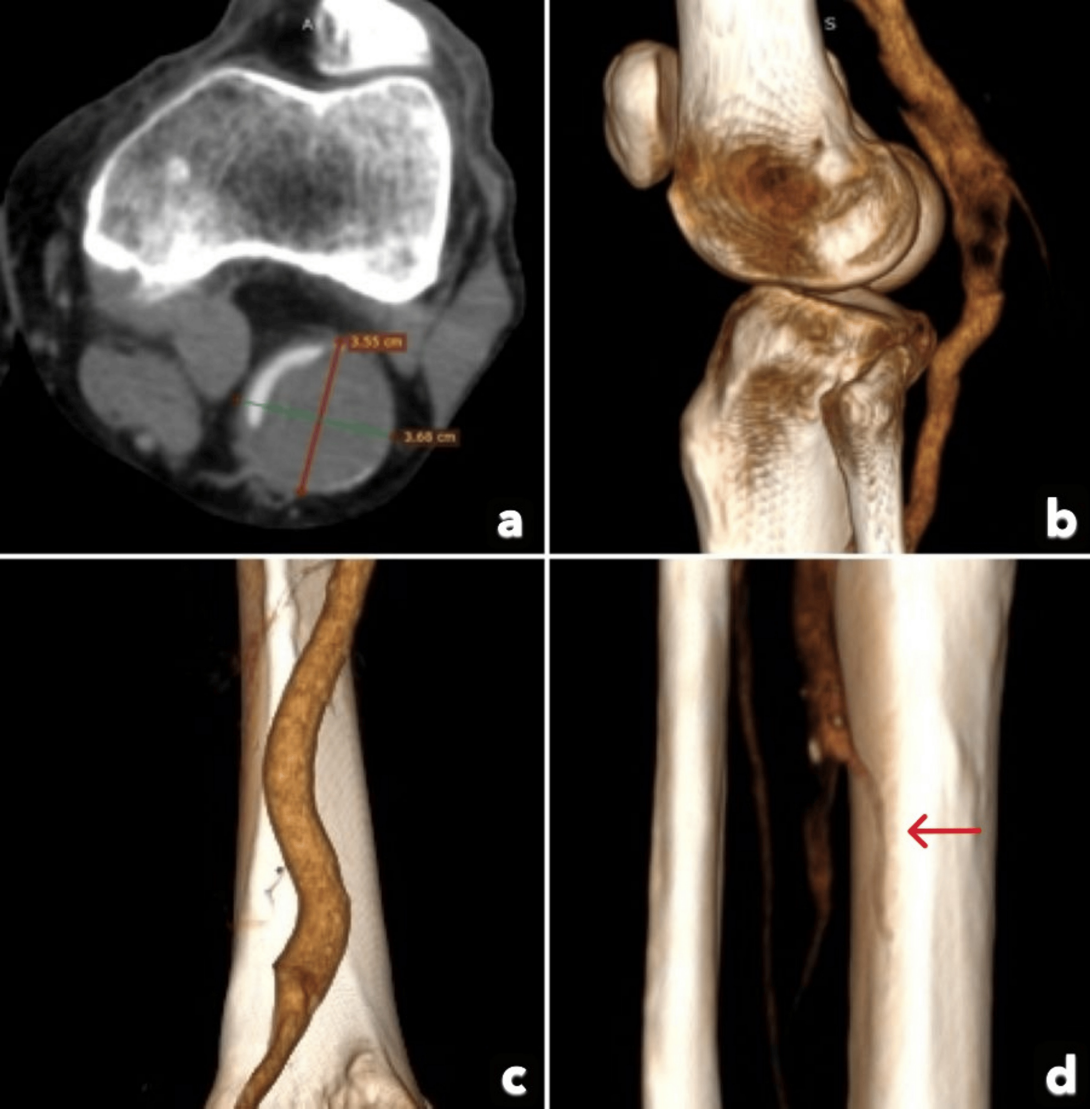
Preoperative CTA showing almost complete thrombosis of the popliteal artery aneurysm (PAA) and no distal vessel run-off Absence of distal vessel run-off due to the thrombosed PAA (red arrow).
